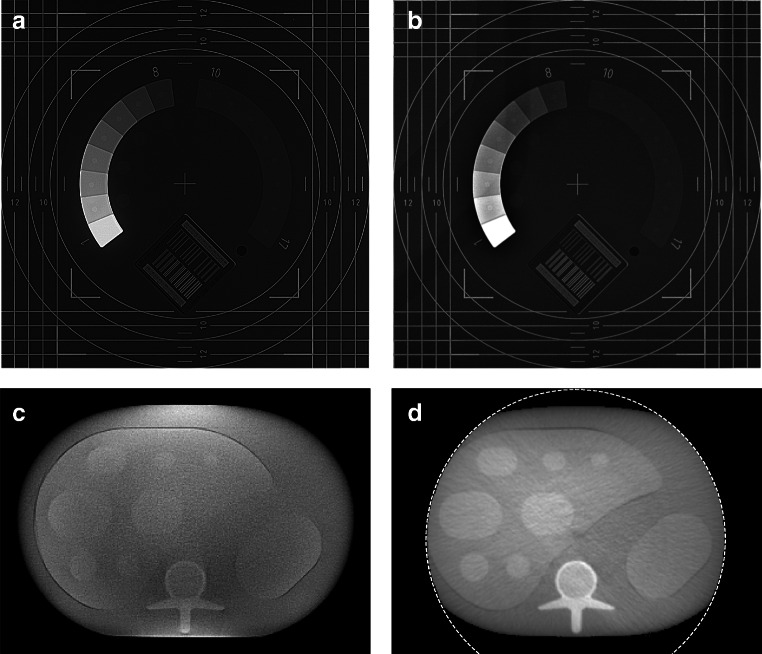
The fluoroscopic image quality evaluation. a The planar fluoroscopic acquisition from IXSI. b The planar fluoroscopic acquisition from the clinical scanner. c The CBCT reconstruction from IXSI. d The CBCT reconstruction from the clinical scanner. The white dashed lines indicate the reconstruction field of view
